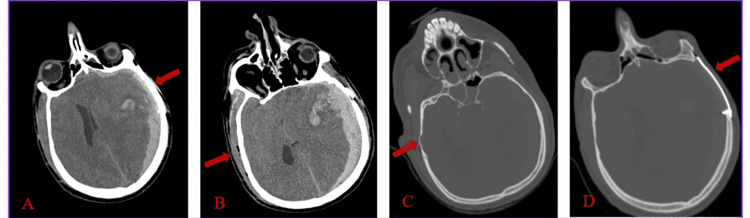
CT brain findings A: left thick acute subdural hematoma with contusion and midline shift (red arrow); B: the air pockets in the subcutaneous tissue of the right side (red arrow); C: bone window shows fracture of the petrous bone on the right side (red arrow); D: bone window shows left side mesh (red arrow)
